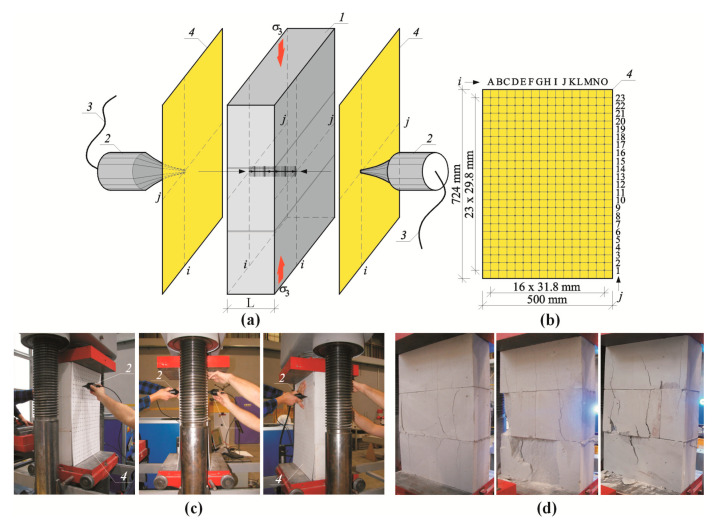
Testing methodology for models made of AAC used in stage II: (a) measurement of velocity of ultrasonic wave propagation at different stress values σ_3_, (b) template geometry used for symmetric arrangement of ultrasonic transducers, (c) models during tests, (d) failure of selected models *1*—masonry units, *2*—ultrasonic transducers, *3*—cables connecting transducers with recording equipment, *4*—templates for symmetric location of ultrasonic transducers.
